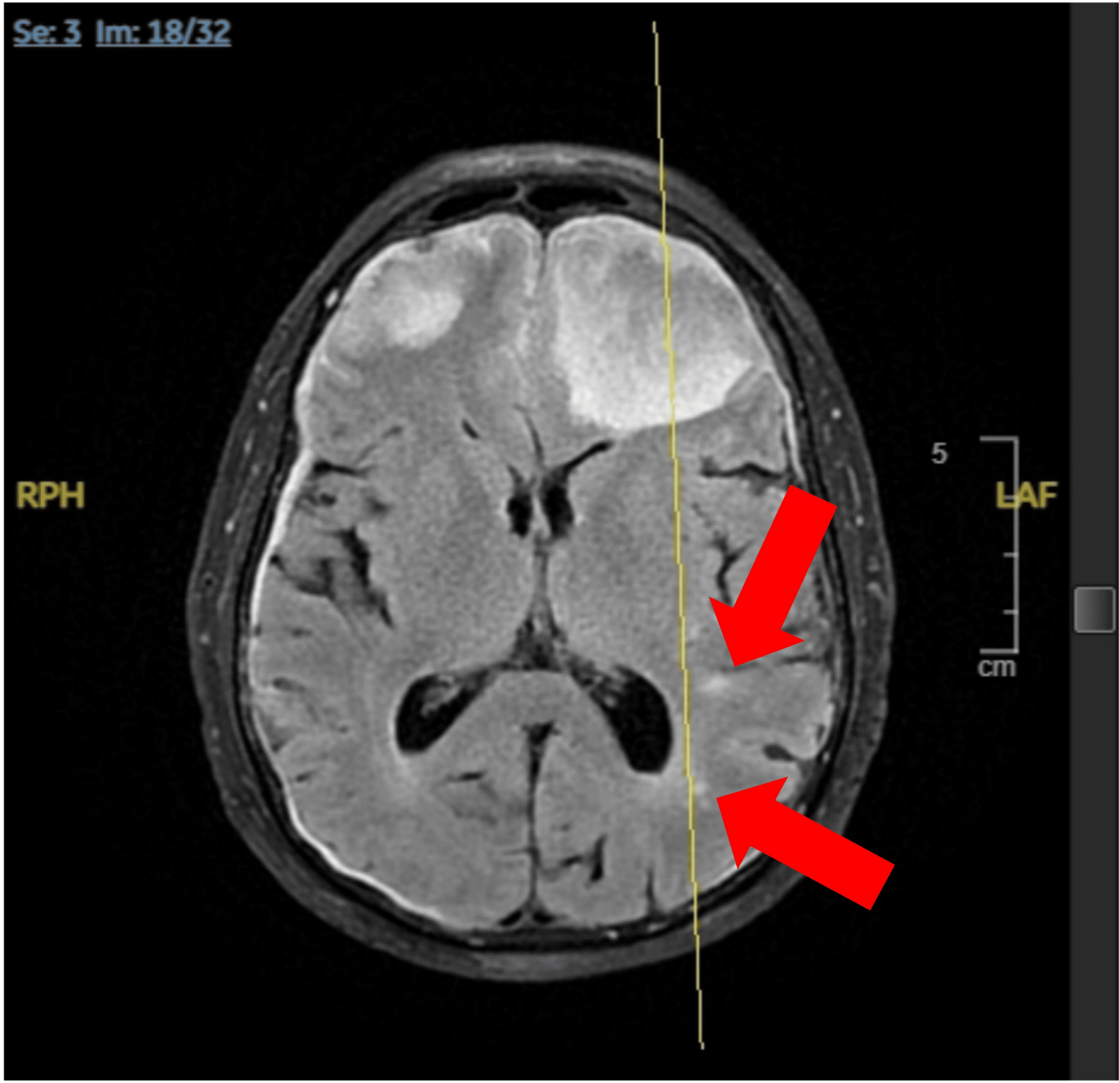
MRI of the brain displaying numerous punctate foci of dural-based nodular enhancement predominantly along the frontal convexities, suspicious for pachymeningeal spread of metastatic disease
